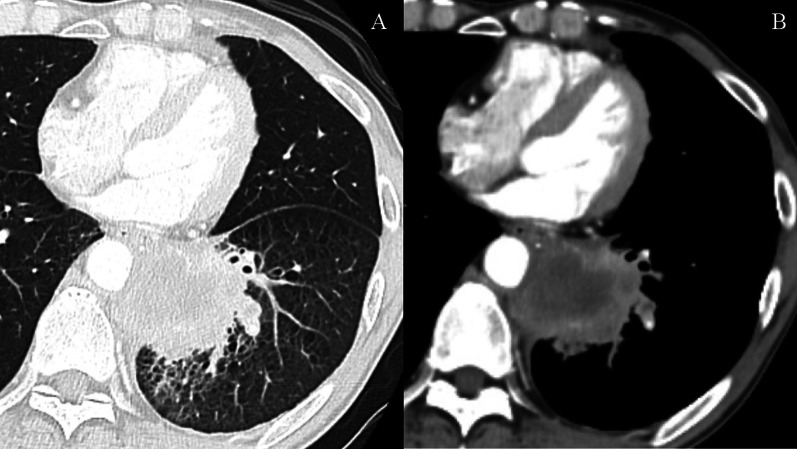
Chest contrast-enhanced CT before neoadjuvant chemoradiotherapy. Chest contrast-enhanced CT showing a huge tumor (diameter; 7 cm) of the left lower lobe of the lung. The tumor was close to the descending aorta, and the invasion to the aortic wall was suspected. CT: computed tomography
